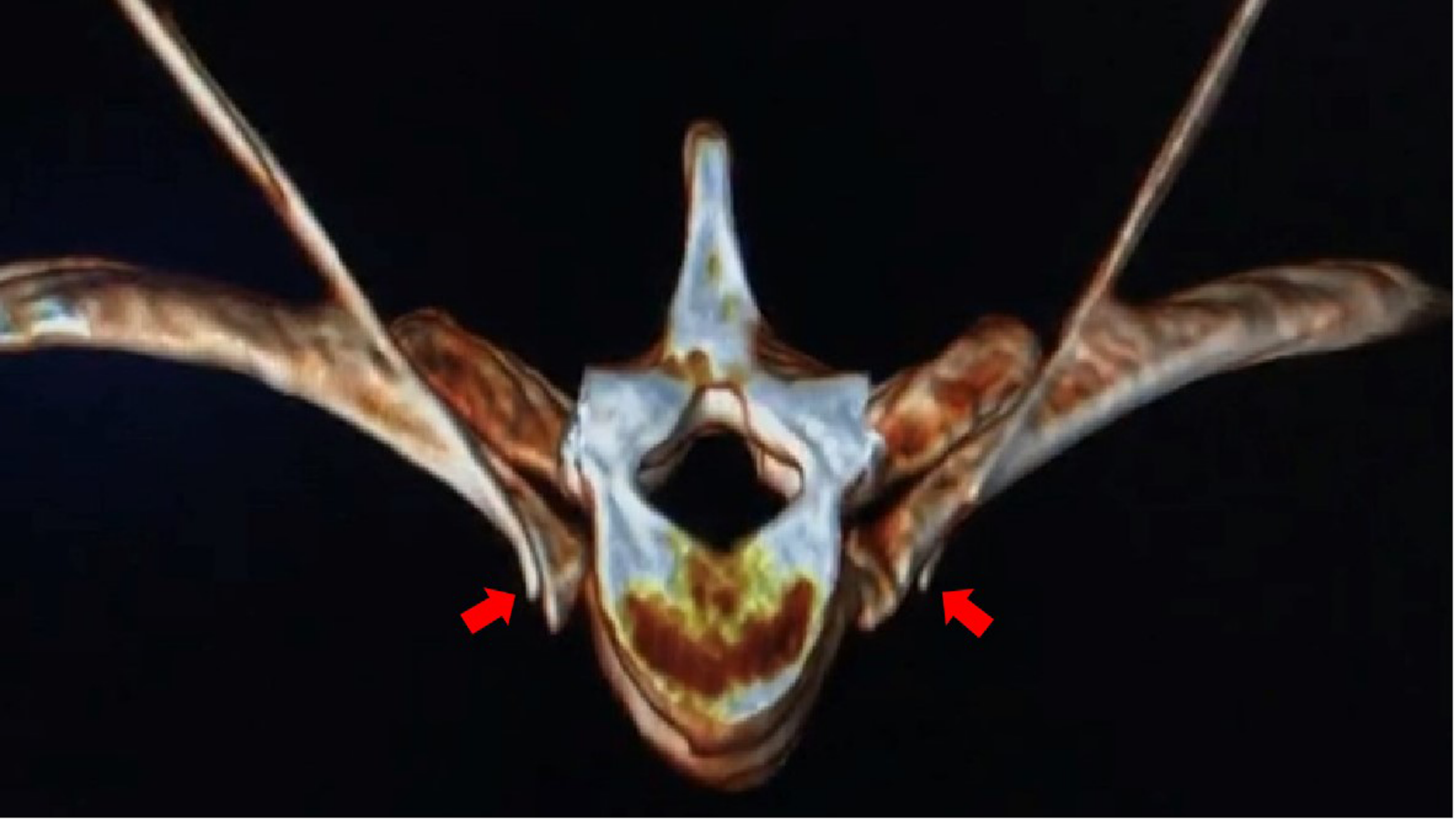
After the three-dimensional reconstruction of CT, the needle tips on both sides reach the anterior superior edge of the 4th rib head and the lateral wall of the T4 vertebral body. (indicated by the red arrow).
